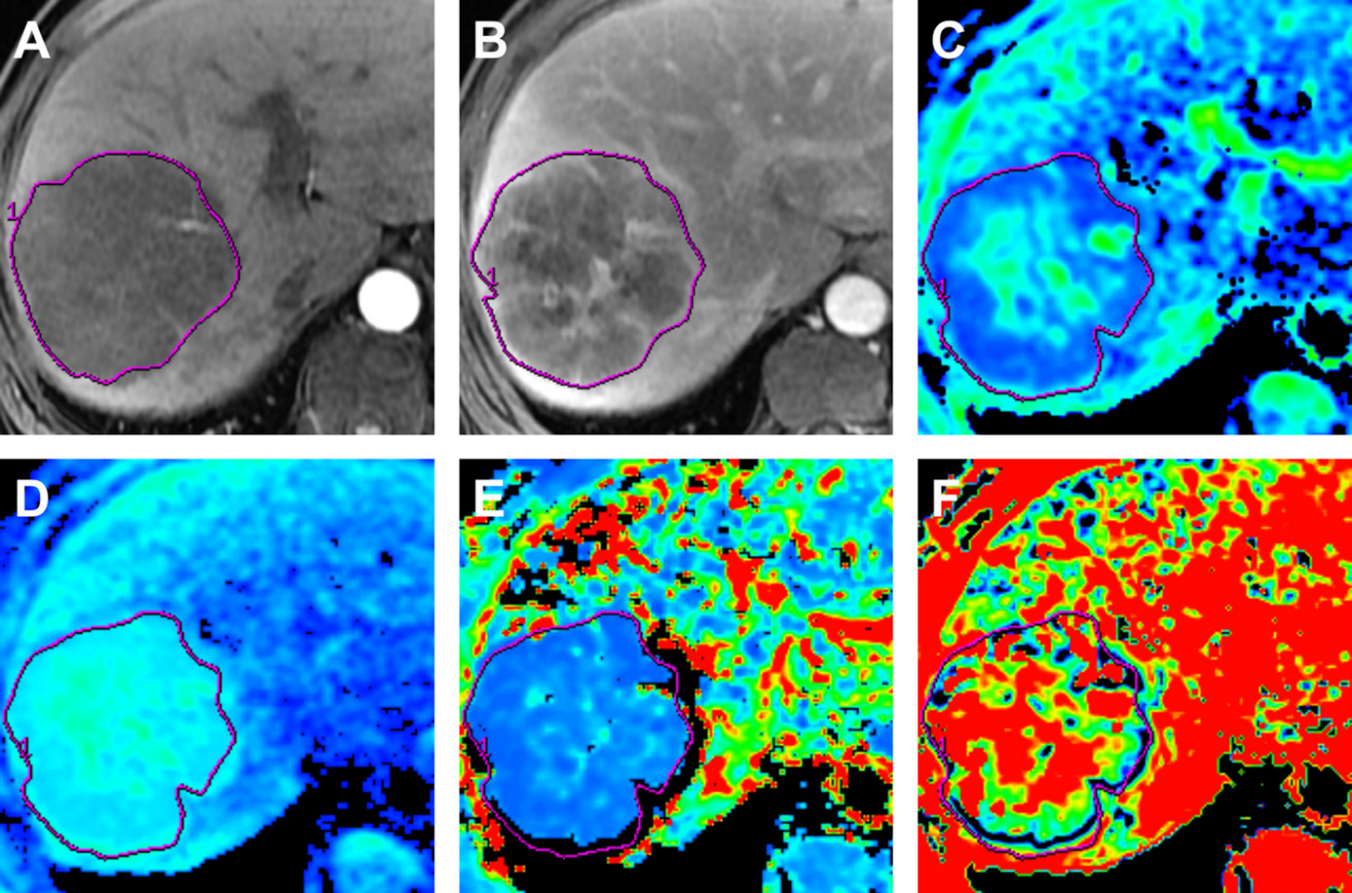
MR images in a 63-year old man with surgically proved ICC (A) Arterial phase MR image. (B) Portal venous phase MR image. (C) Standard ADC map. (D) ADC_slow_ map. (E) ADC_fast_ map. (F) *f* map. The tumor shows peripheral rim-like enhancement on arterial phase MR image and with progressive central enhancement on portal venous phase. The ADC map demonstrates a higher ADC value (1.41 × 10^−3^ mm^2^/s) compared with cutoff value (1.18 × 10^−3^ mm^2^/s), the ADC_slow_ also demonstrates a higher ADC value (1.26 × 10^−3^ mm^2^/s) compared with cutoff value (1.06 × 10^−3^ mm^2^/s).
